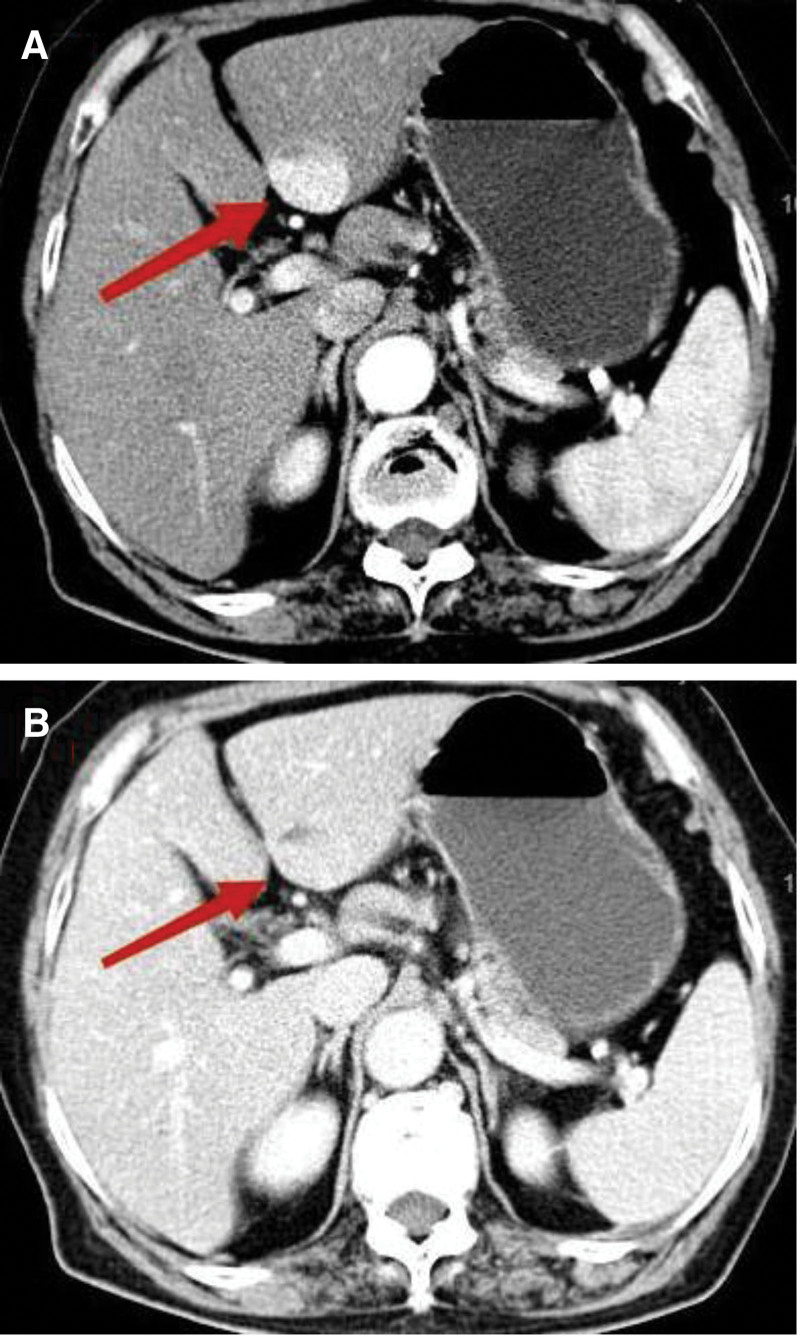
(A) CT contrast-enhanced arterial phase shows significant enhancement of the left lateral lobe of the liver, with patchy suspicious fatty density; (B) venous phase shows similar enhancement to the liver parenchyma. CT = computed tomography.
